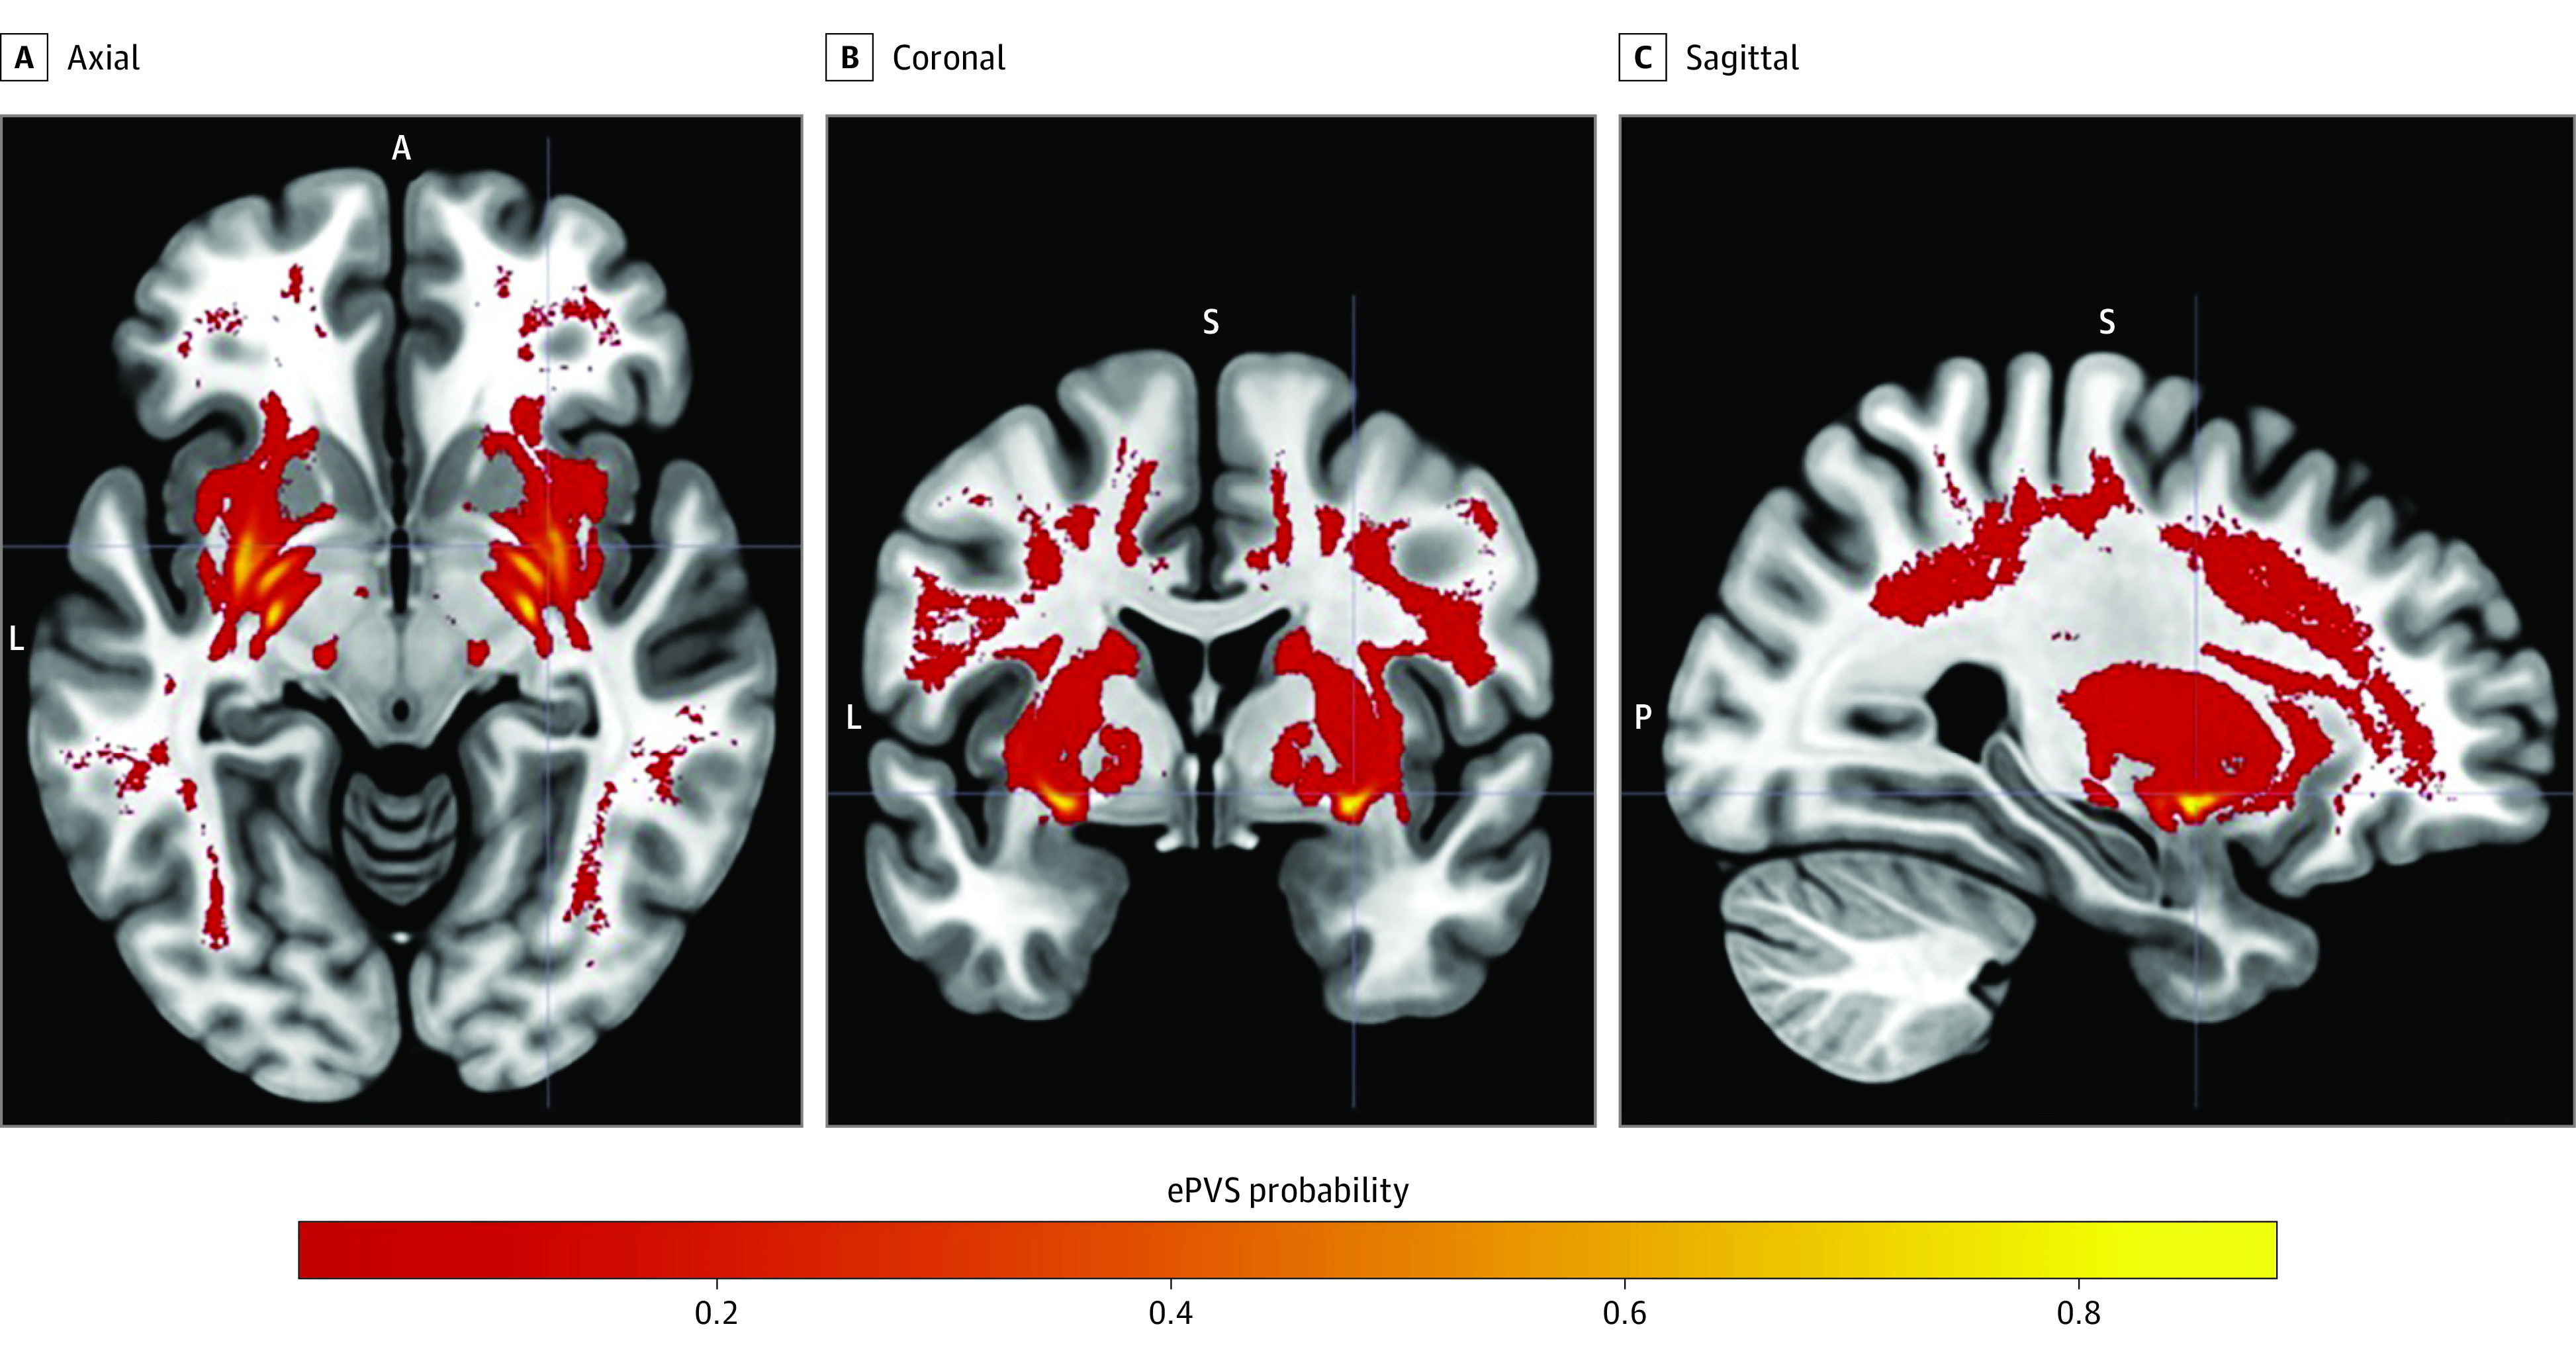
Frequency Maps Depicting the Distribution of Enlarged Perivascular Spaces (ePVSs) Values represent the probability of detecting an ePVS at the respective anatomic location. A indicates anterior; FLAIR, fluid-attenuated inversion recovery; L, left; P, posterior; and S, superior.
